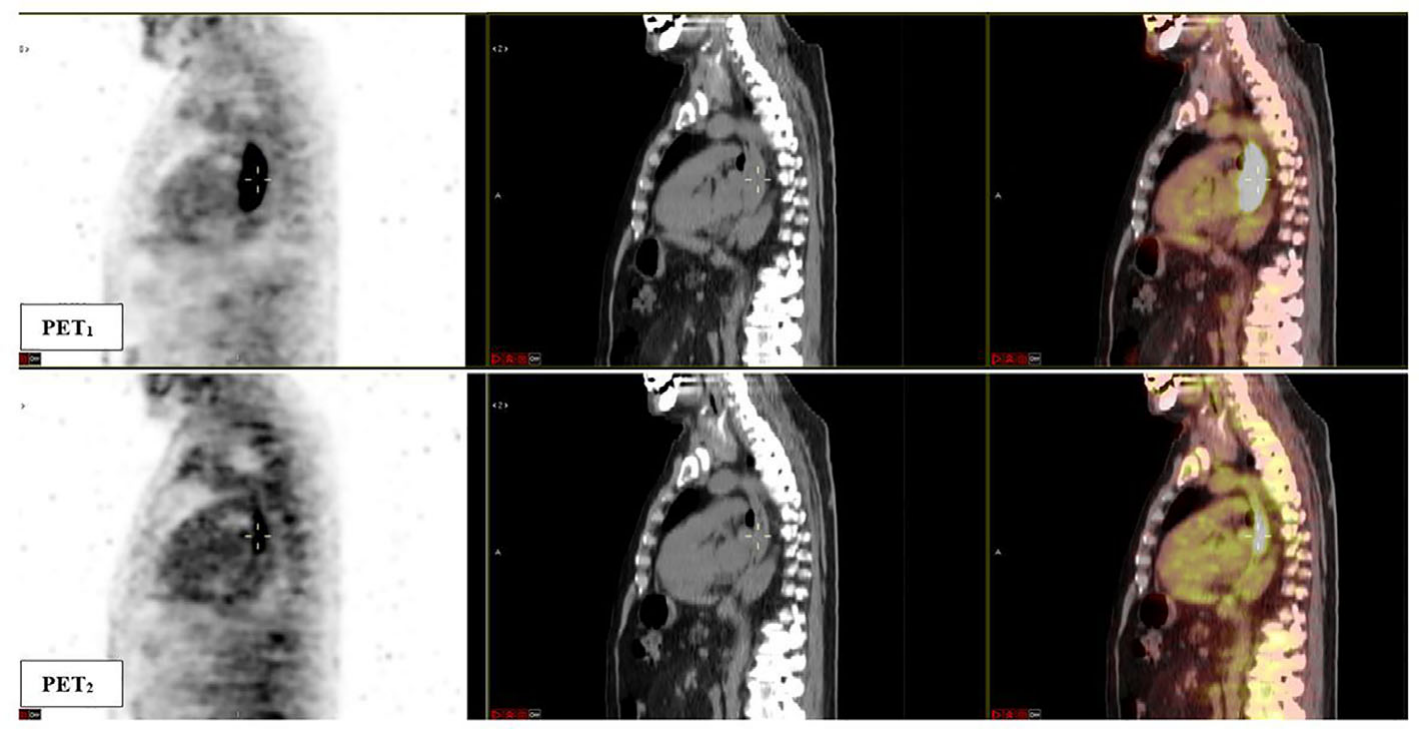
Sagittal fused ^18^F-FDG PET/CT images obtained at baseline (PET_1_) and after induction chemotherapy (PET_2_). A significant response to induction chemotherapy (reduction in metabolic parameters) of the esophageal lesion can be observed. The patient was classified as TRG1 at final pathological examination. PET_1_ parameters: SUV_max_ 26.9, SUV_mean_ 12.7, MTV 43.7 ml, TLG 553.9; PET_2_ parameters: SUV_max_ 6.7, SUV_mean_ 3.2, MTV 15.9 ml, TLG 50.5.
